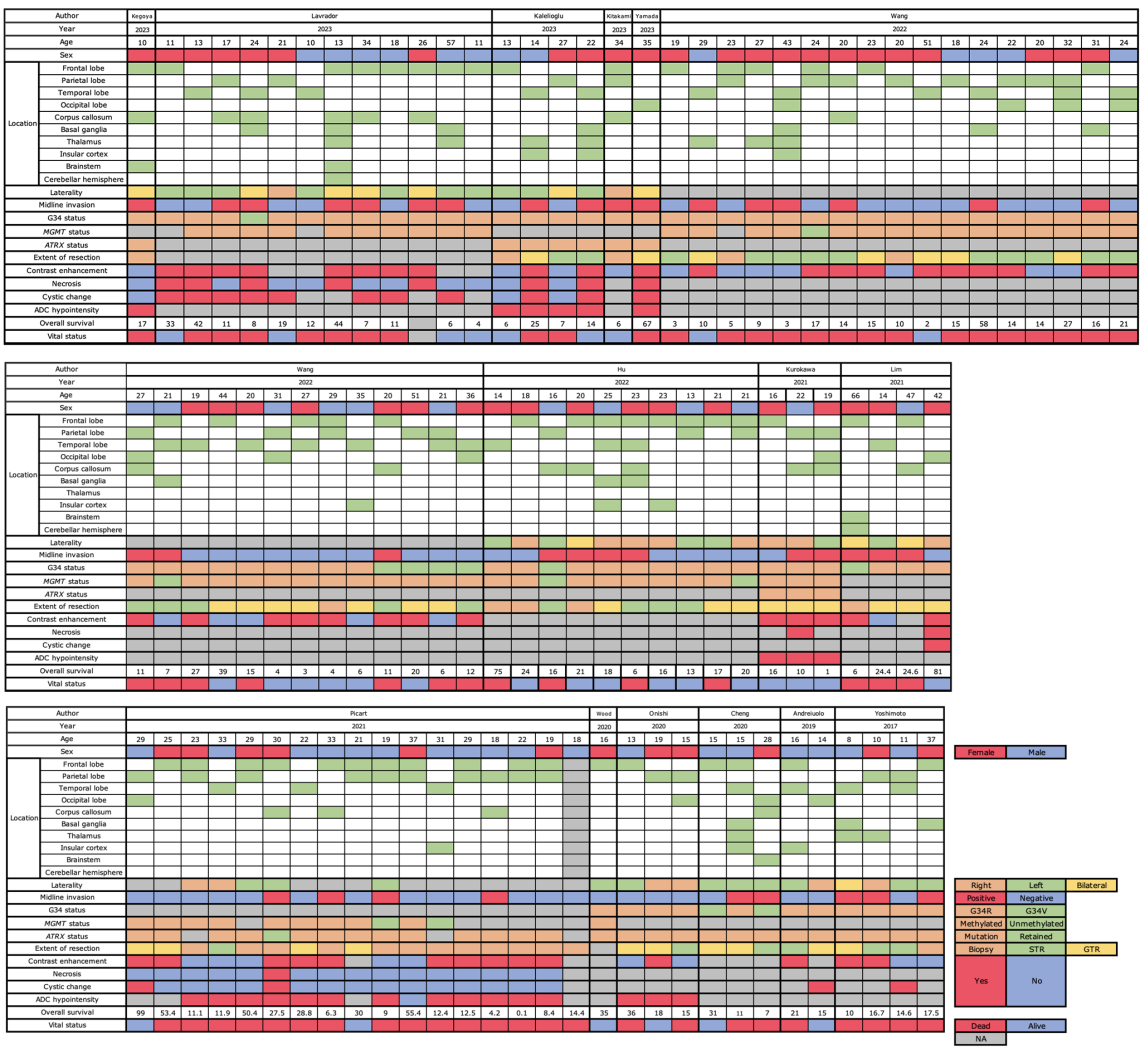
Summary of the clinical, radiographic, and genetic features in patients with diffuse hemispheric gliomas, H3 G34-mutant cases. ADC: apparent diffusion coefficient
